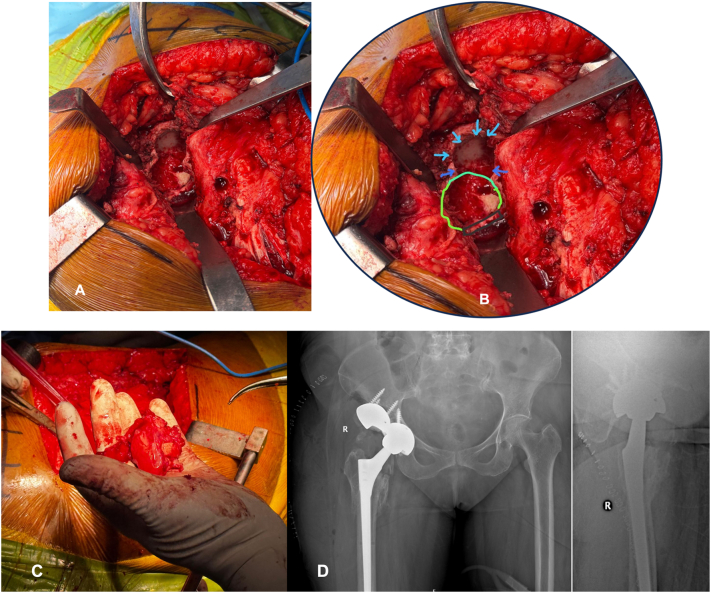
A): Clinical Intra operative image showing acetabular erosions. B) Close up view with markings – Blue arrow mark pointing to the acetabular erosion by cephalic screw, green depicts acetabular margin, black line – Transverse Acetabular ligament (TAL). C: Femoral head with complete delamination of cartilage and sclerotic. D: Post op radiograph AP and Lateral view showing total Hip arthroplasty with acetabular augments.
